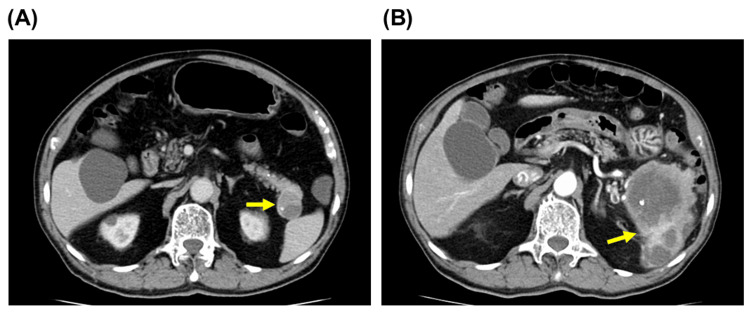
Contrast-enhanced CT images of the pancreatic tumor (A) Initial detection revealed a cystic lesion with calcification measuring 30 mm in the pancreatic tail (arrow). (B) At one year and eight months follow-up, significant enlargement and infiltration into the spleen were observed (arrow).
